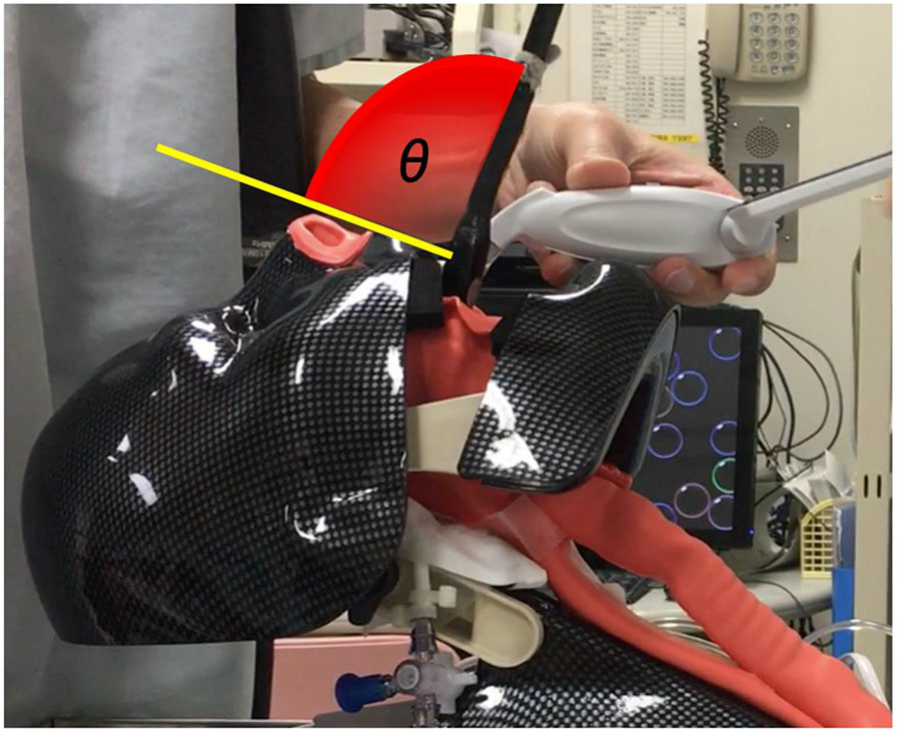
The angle θ corresponds to the insertion angle of the TEE probe. The angle θ is defined as that between the TEE probe and the lip-nose tip line (yellow line) when the probe passes through the lips. TEE, transthoracic echocardiography
